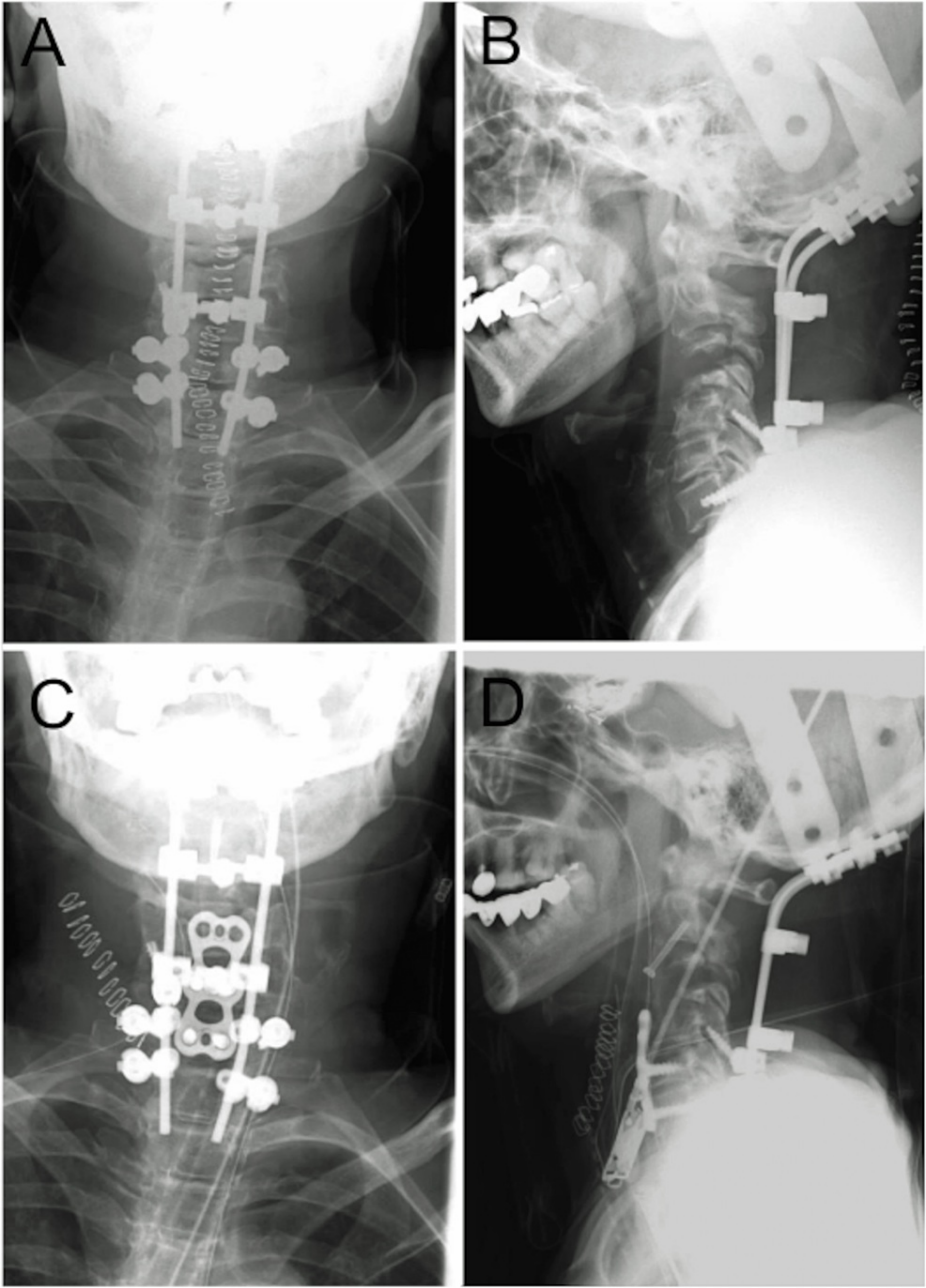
X-ray Images Post-First and Second Operations Cervical alignment achieves correction through posterior fixation extending from the occipital bone to Th1 (A, B). Anterior cervical alignment is supported through anterior fixation utilizing a canulated mini screw to secure C2 and a fibula graft, in addition to a titanium plate for fixation of the graft bone and the vertebral bodies of C5 and C6 (C, D).
